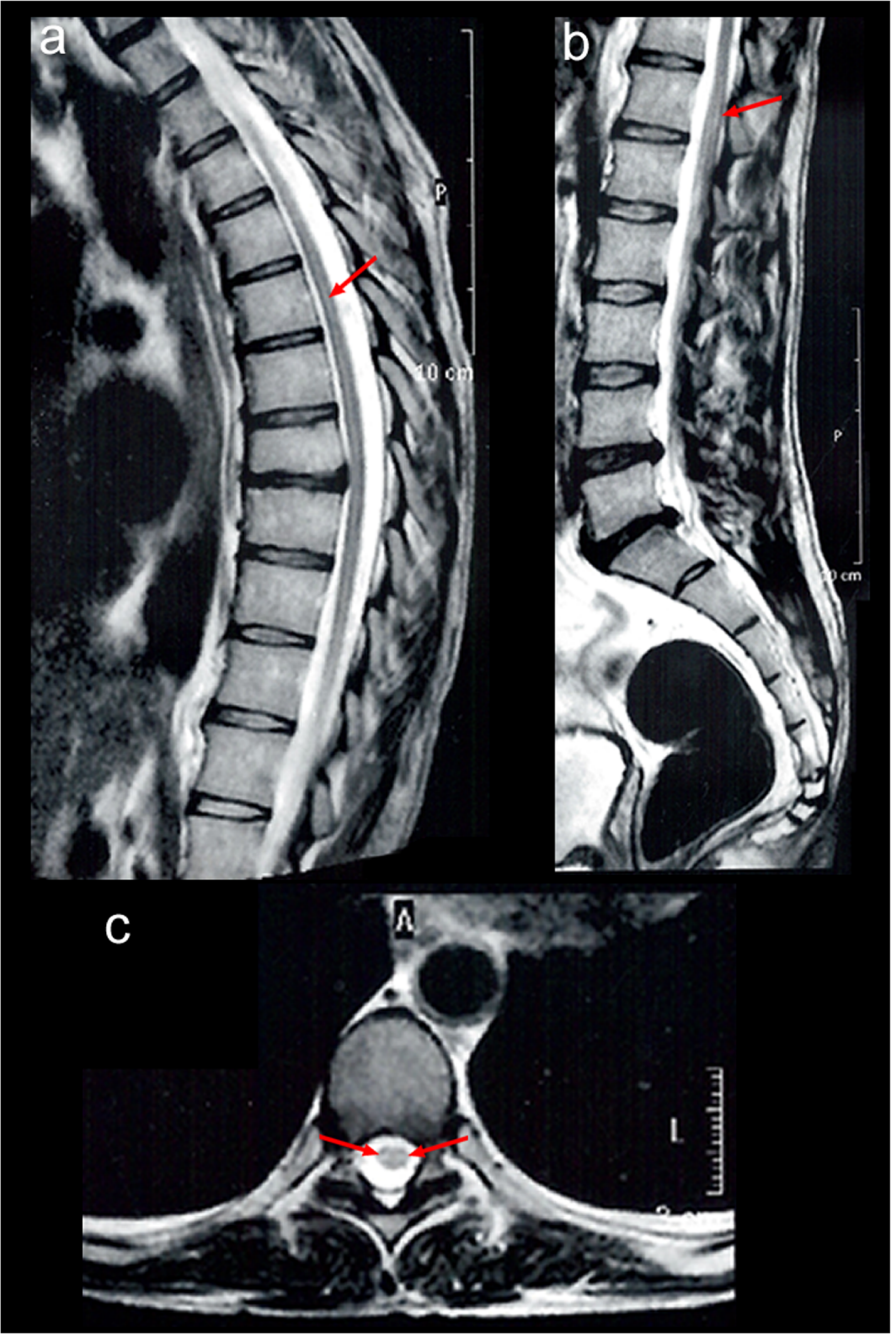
MRI of the thoracic and lumbosacral column of patient 1. a/b) T2 sagittal sections hyperintensity in thoracic and lumbosacral cord without gadolinium contrast enhancement. c) T2 axial sections hyperintensity in thoracic and lumbosacral cord without gadolinium contrast enhancement
